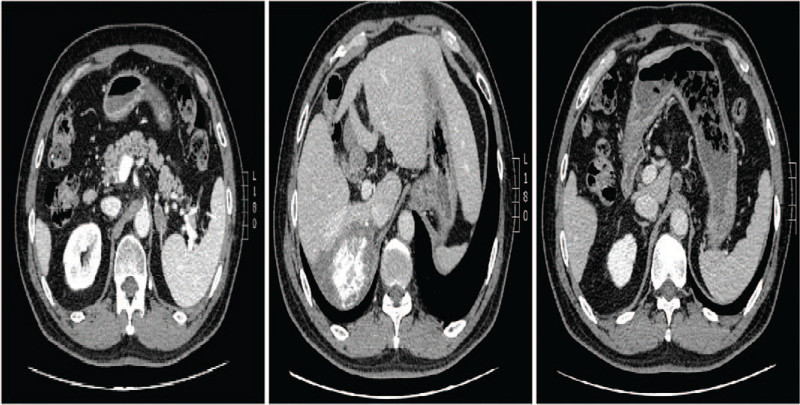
In April, 2020, enhanced CT showed that the left adrenal gland metastasis recurred, the lymph nodes of the hepatic hilar and retroperitoneal were enlarged. CT = computed tomography.
